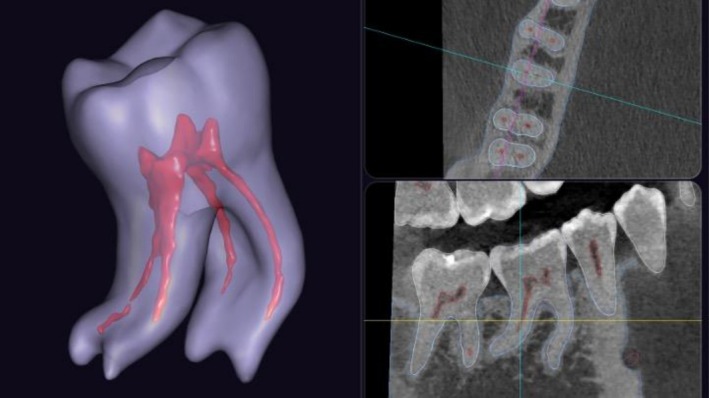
Screenshots of the investigated mandibular molar in segmented view by Diagnocat (left) and corresponding annotated CBCT slices (right).
